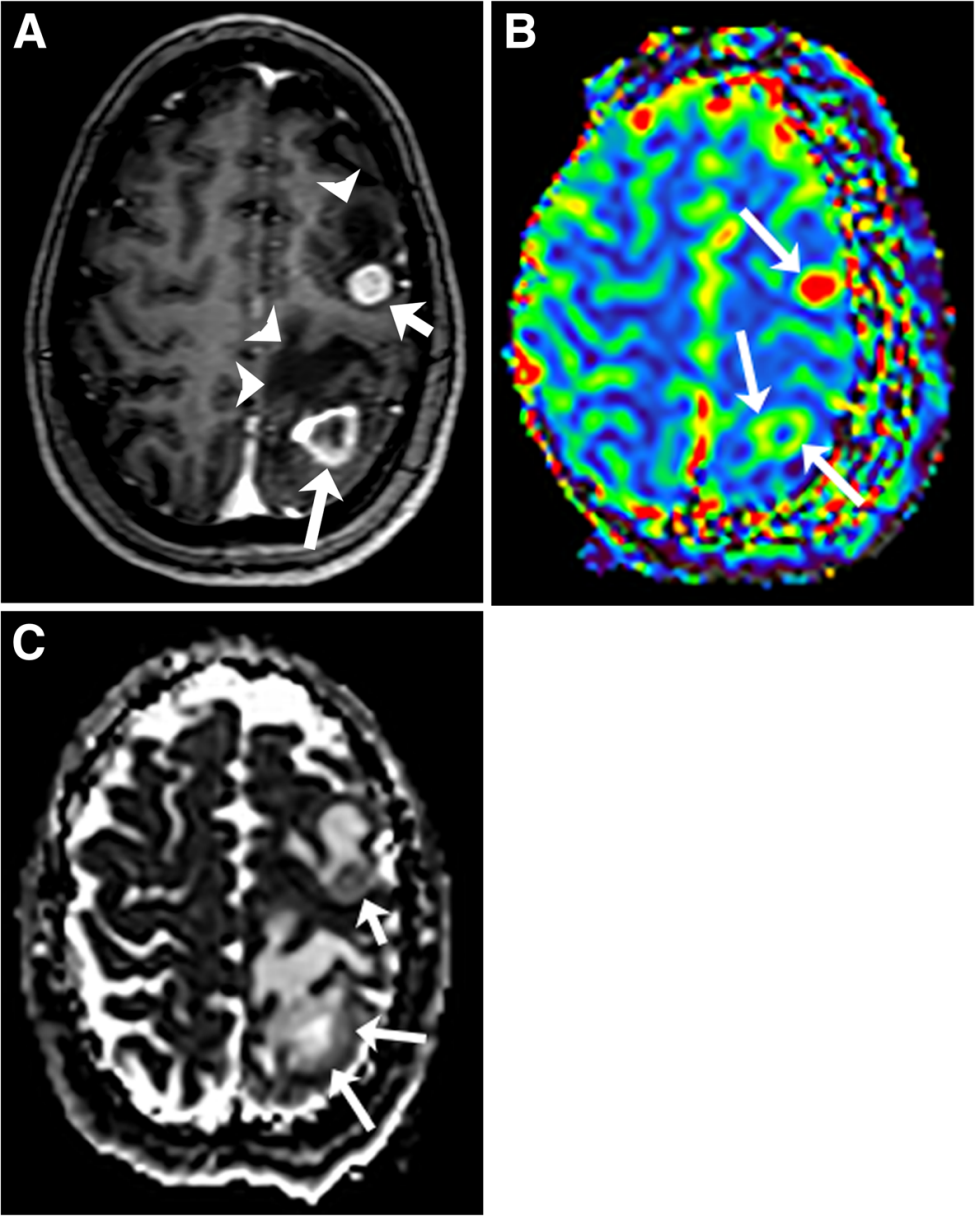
Metastasis in a patient with lung adenocarcinoma. T1-weighted image with gadolinium (a) demonstrates a ring-enhancing left parietal lobe mass (long arrow) and a solidly enhancing left frontal lobe mass (short arrow). Extensive vasogenic edema is around each of these lesions (arrowheads). On CBV map (b), the CBV is elevated in the enhancing components (arrows). No increased peri-tumoral enhancement or elevated perfusion is present. ADC map (c) shows minimally decreased ADC in the parietal mass (long arrows) and mildly decreased ADC in the frontal mass (short arrow)
